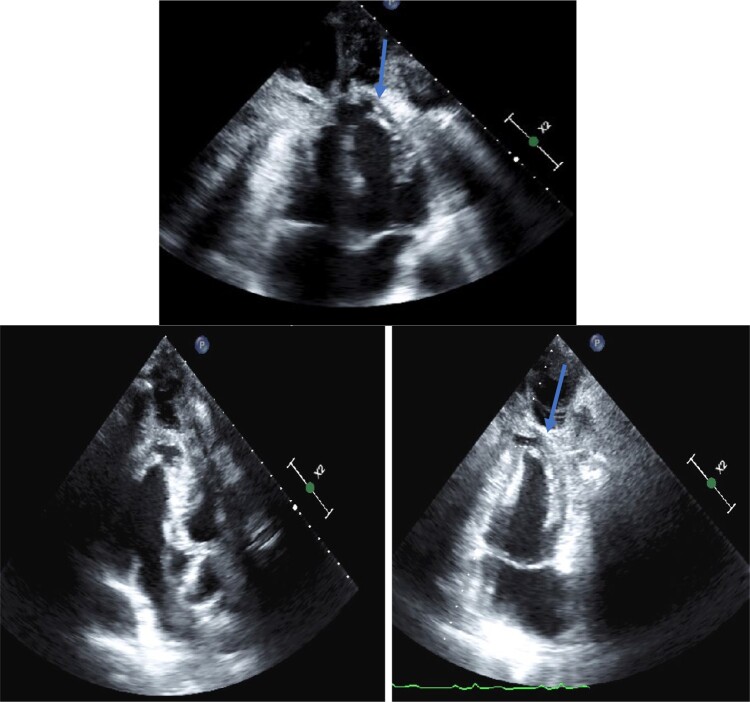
Displays a set of three views of the transthoracic echocardiogram, including a four-chamber view, a two-chamber view, and a three-chamber view. The images reveal a localized thickening of the apical left ventricular wall (arrow), which corresponds to the site of tumour attachment. The thickening is visualized as a focal area of hyperechogenity, with a blurred margin and a heterogenous texture.
